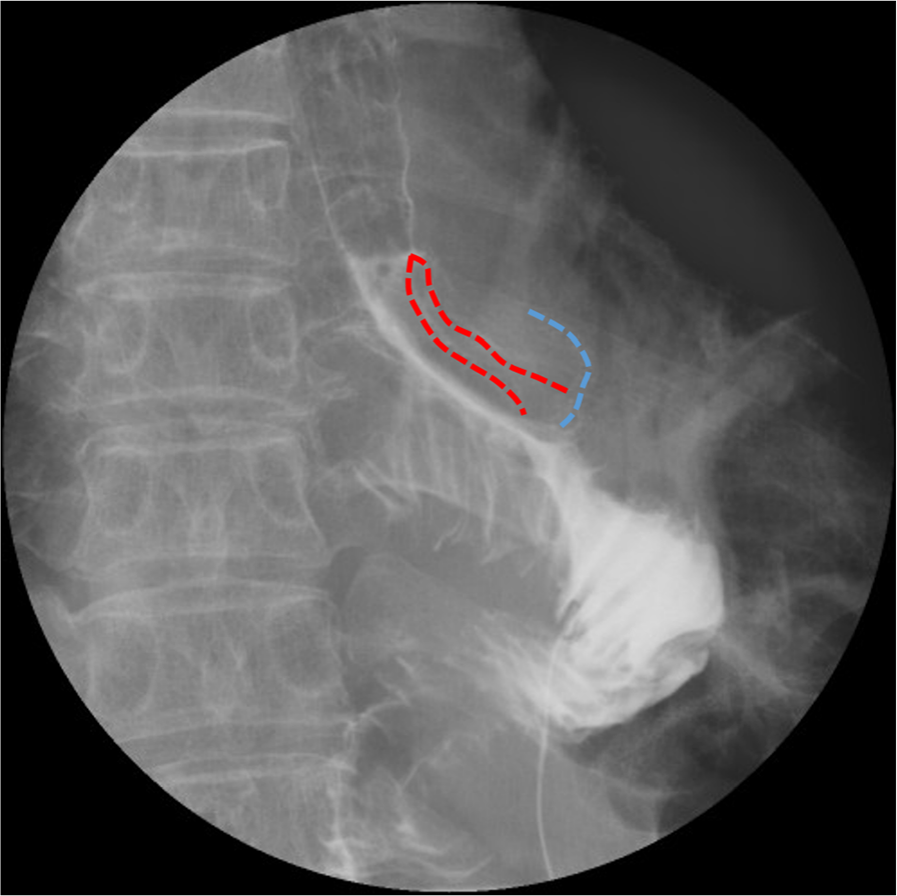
X-ray image with contrast medium on postoperative day 2 revealing no leakage of the esophagojejunostomy. Esophageal stump (dashed blue line) and stapled lines of the entry hall (dashed red line) can be seen
